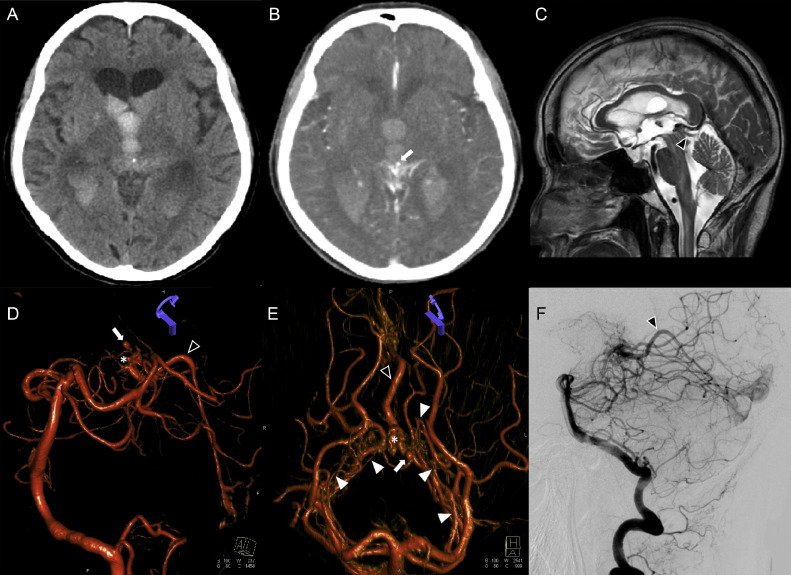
(A) Computed tomography revealed intraventricular hemorrhage and hydrocephalus. (B) Computed tomography angiography revealed an aneurysm in the region of the posterior third ventricle. (C) Sagittal T2-weighted magnetic resonance image demonstrates a flow void around the pineal body draining into the confluence of sinuses (black arrowhead). (D) Lateral view on digital subtraction angiography reveals arteriovenous malformation of the pineal region with a small nidus (asterisk), a 2 mm distal flow-related aneurysm (white arrow), and dilated veins draining into the confluence of sinuses (black arrowhead) (E) Anterior-posterior view on digital subtraction angiography reveals arteriovenous malformation of the pineal region with a small nidus (asterisk) fed by the posterolateral choroidal artery (white arrowhead) and dilated veins draining into the confluence of sinuses (black arrowhead). (F) Lateral view on digital subtraction angiography reveals dilated veins draining into the confluence of sinuses (black arrowhead).
